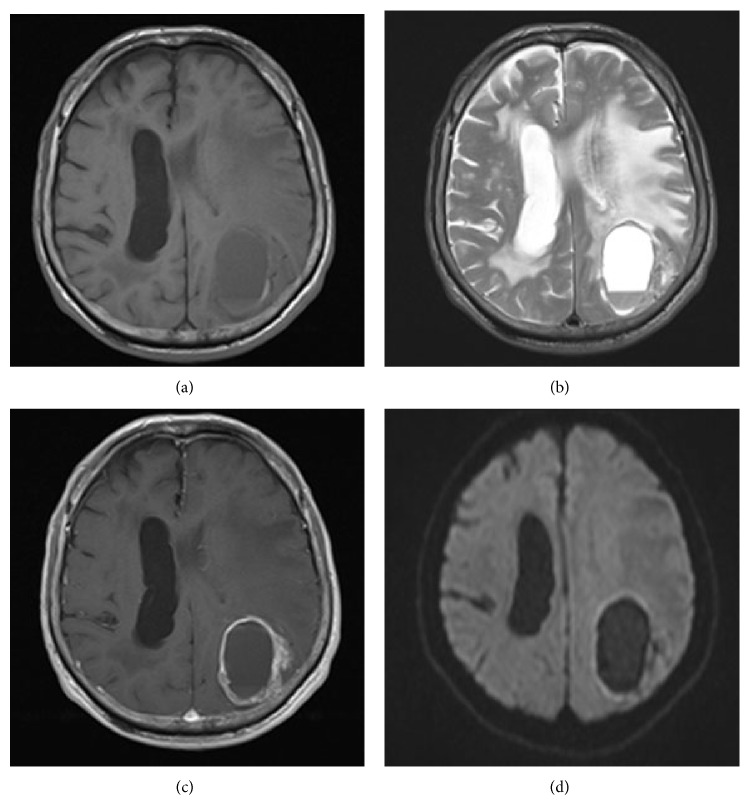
(a) Axial plain T1-weighted MR image shows homogeneous low-intensity area in the left temporal and parietal lobes. (b) Axial plain T2-weighted MR image shows a high-intensity area that indicates hemorrhage, a cyst, and marked peritumoral edema. (c) Diffusion-weighted image (DWI) shows low-intensity area not indicative of abscess. (d) Axial enhanced T1-weighted MR image shows ring enhancement.
